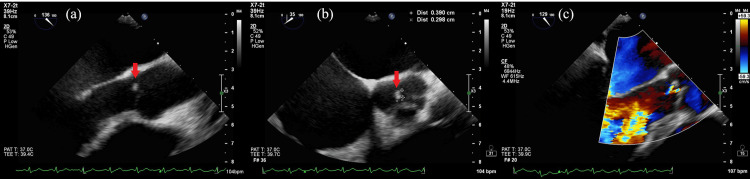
Transesophageal echocardiography at the beginning of treatment revealing vegetation on the aortic valve (red arrow) (a) (b) and mild aortic regurgitation (c)
